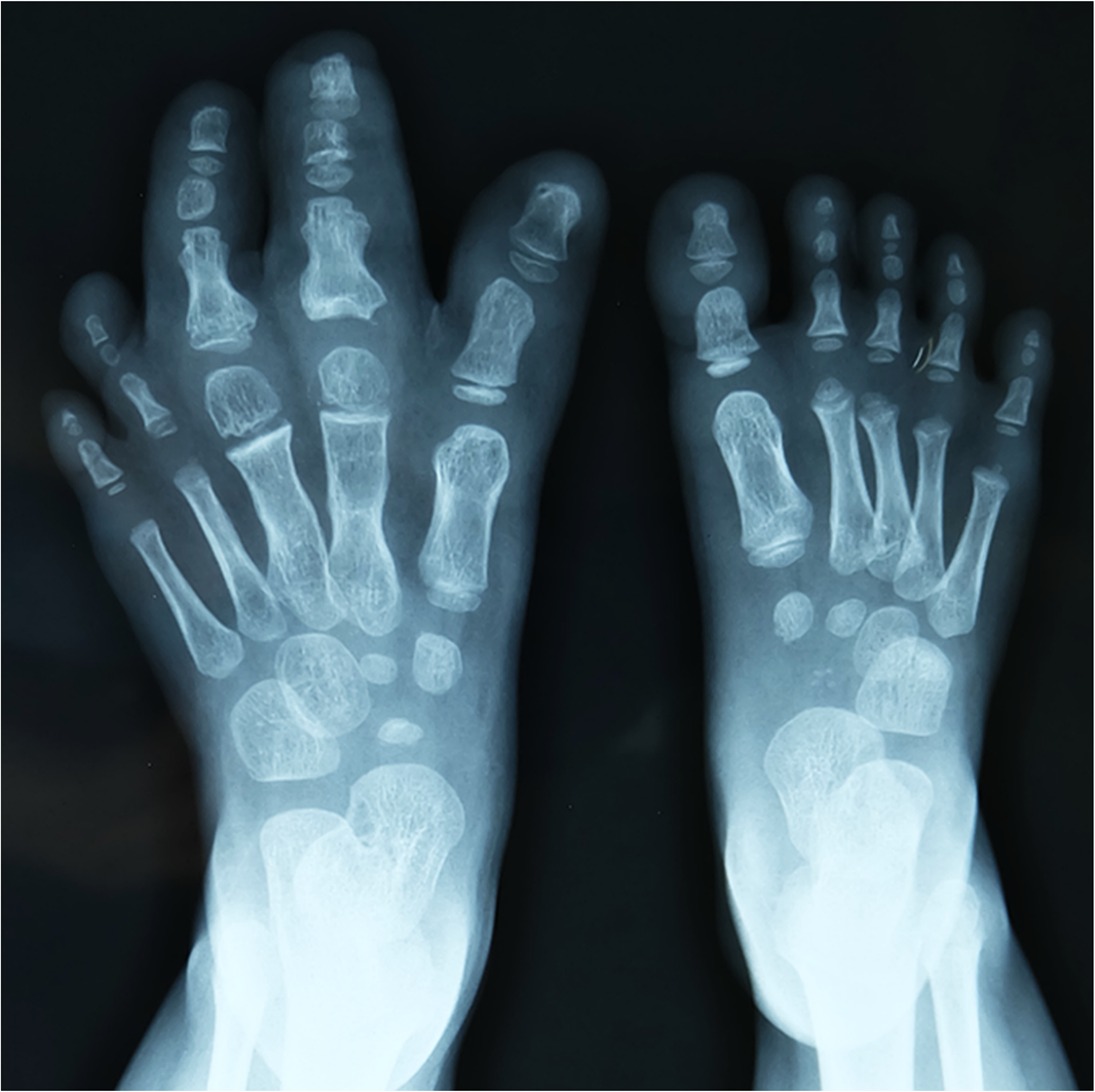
Plain radiograph showing hypertrophy of phalanges and metatarsal bones in the first, second, and third ray as well as advanced bone maturation in the second and third metatarsal capital epiphyses. The phalangeal basal epiphyses were well formed and larger than the normal foot
